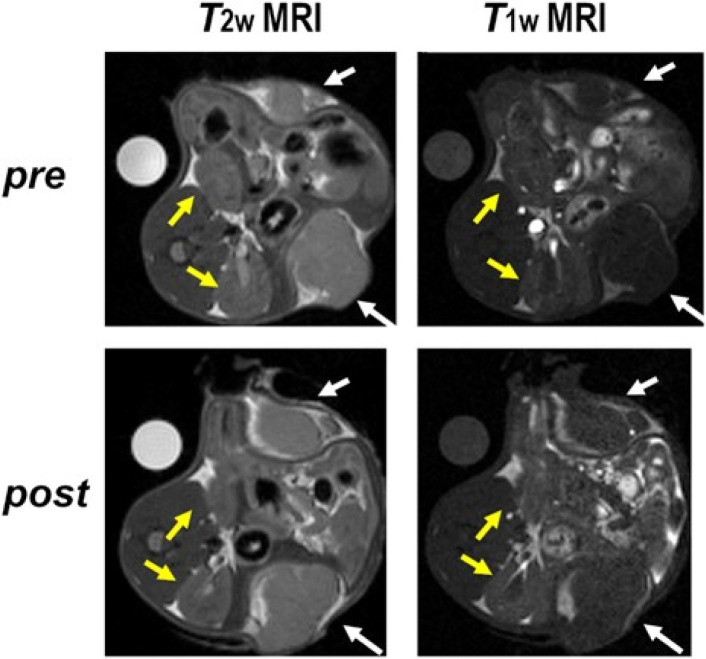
Representative *T*_2w_ and *T*_1w_ MR images pre and post (*t* = 15 min) i.p. administration of PEG/Fe(iii)–GA showing tumours (white arrows) and kidneys (yellow arrows).
